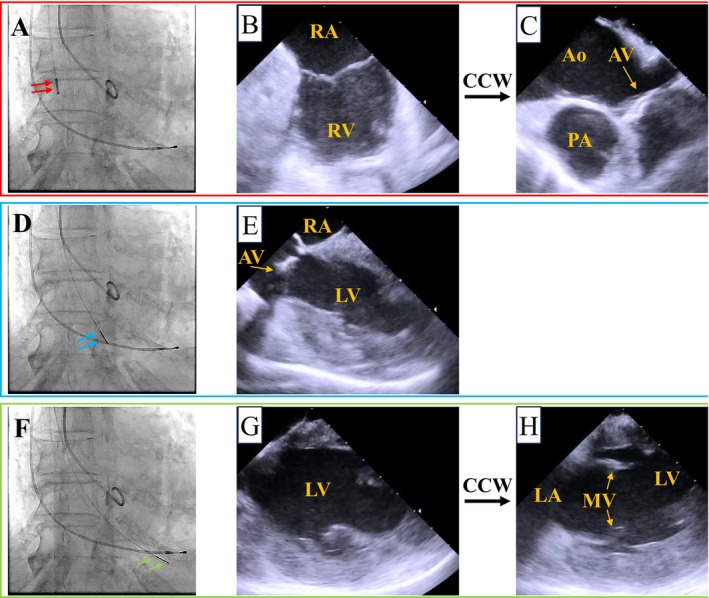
Location of ICE catheter and images obtained at that location. (A) ICE catheter (red arrow) at the right atrium in fluoroscopic view. (B) Long‐axis tricuspid valve view with the catheter at the right atrium. (C) Long‐axis ascending aorta and aortic valve view with the catheter at the right atrium. This view is obtained by counterclockwise rotation from the long‐axis tricuspid valve view. (D) ICE catheter (blue arrow) near the tricuspid valve at the right atrium in fluoroscopic view. (E) Long‐axis left ventricular outflow tract view with the catheter near the tricuspid valve at the right atrium. (F) ICE catheter (green arrow) at the right ventricle in fluoroscopic view. (G) Long‐axis left ventricle view with the catheter at the right ventricle. (H) Long‐axis mitral valve view with the catheter at the right ventricle. This view is obtained by counterclockwise rotation from the long‐axis left ventricle view. Ao, aorta; AV, aortic valve; CCW, counterclockwise; ICE, intracardiac catheter echocardiography; LA, left atrium; LV, left ventricle; MV, mitral valve; PA, pulmonary artery; RA, right atrium; RV, right ventricle.
